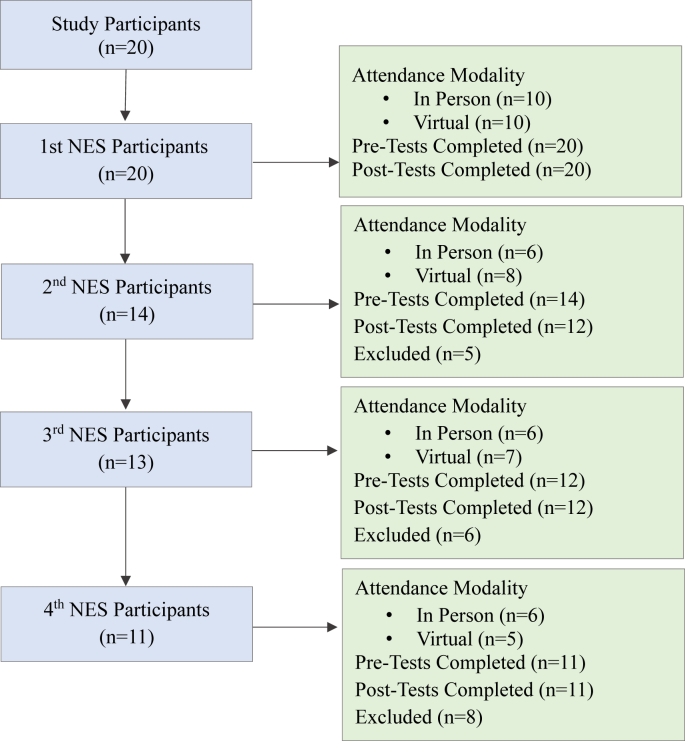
FOOD4MOMS participants’ attendance by nutrition education session and mode of participation. Participants were Hispanic pregnant women in Hartford, CT. NES, nutrition education session;
